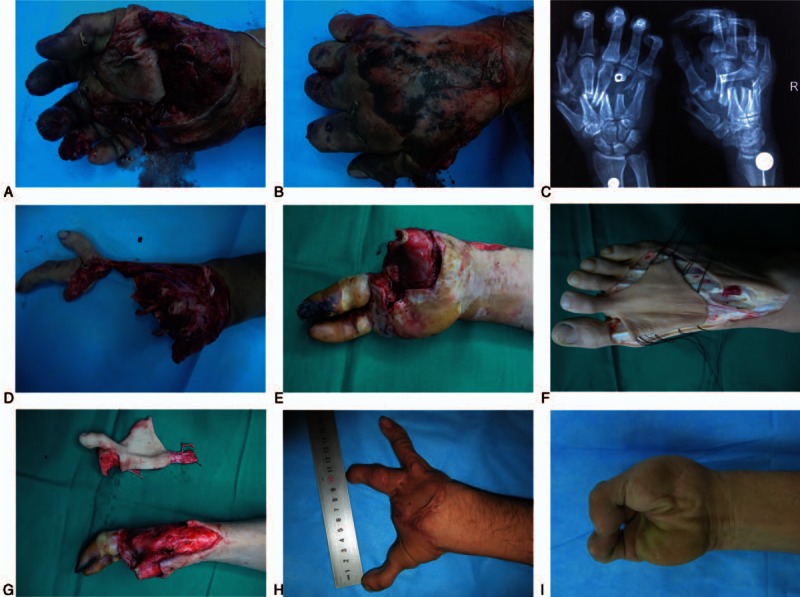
Preoperative, intraoperative, and follow-up pictures of patient 5. Picture of the injured hand showing the extent of (A and B) the injury; (C) the X-ray radiograph of the right hand; (D) the wound after debridement; (E) the surviving ring and little fingers; (F and G) dissection of the second toe with dorsal pedis artery flap; and (H and I) the results after 25 mo.
